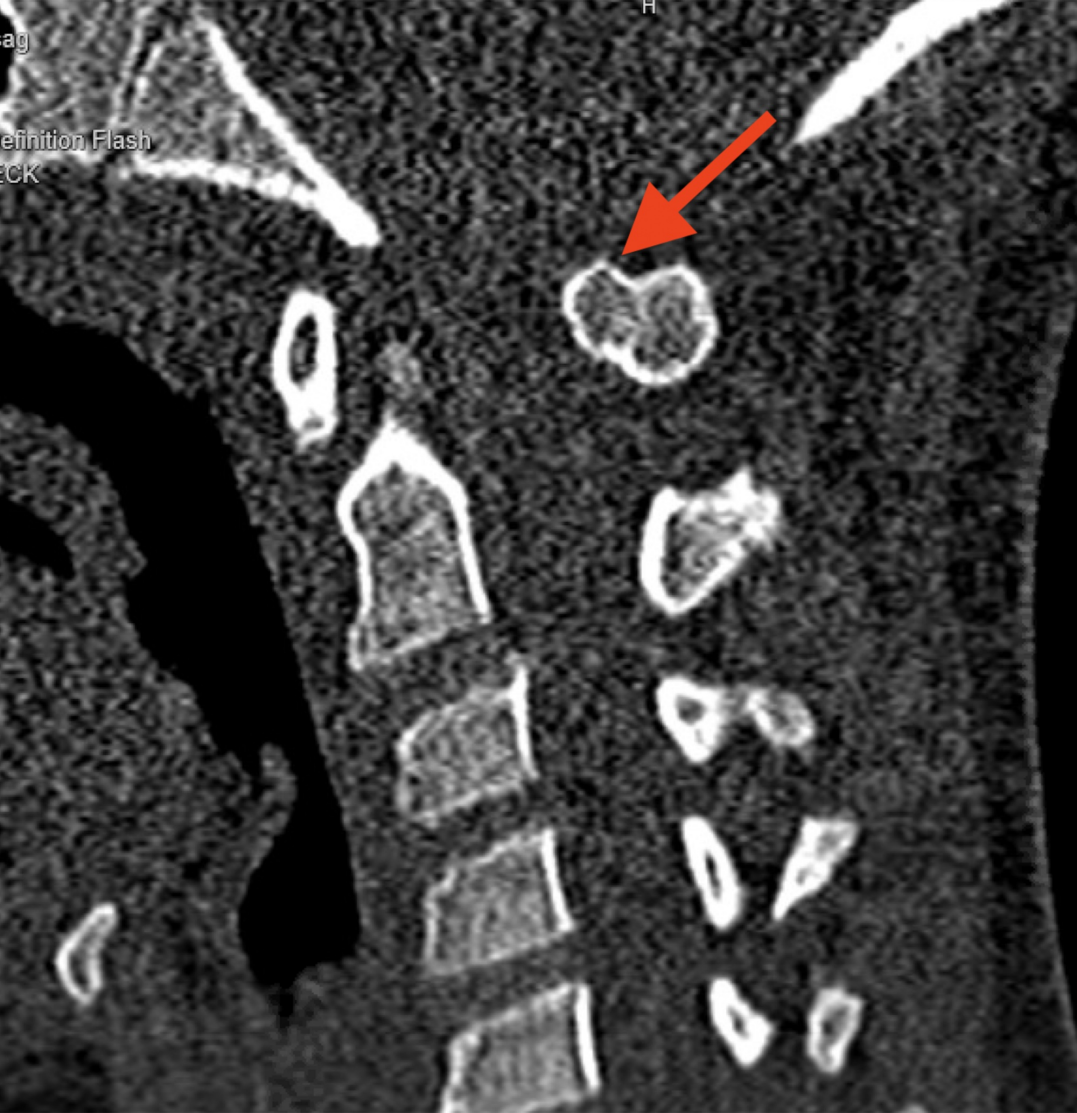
Cervical CT sagittal view demonstrating a pedunculated osteochondroma on the posterior arch of C1
